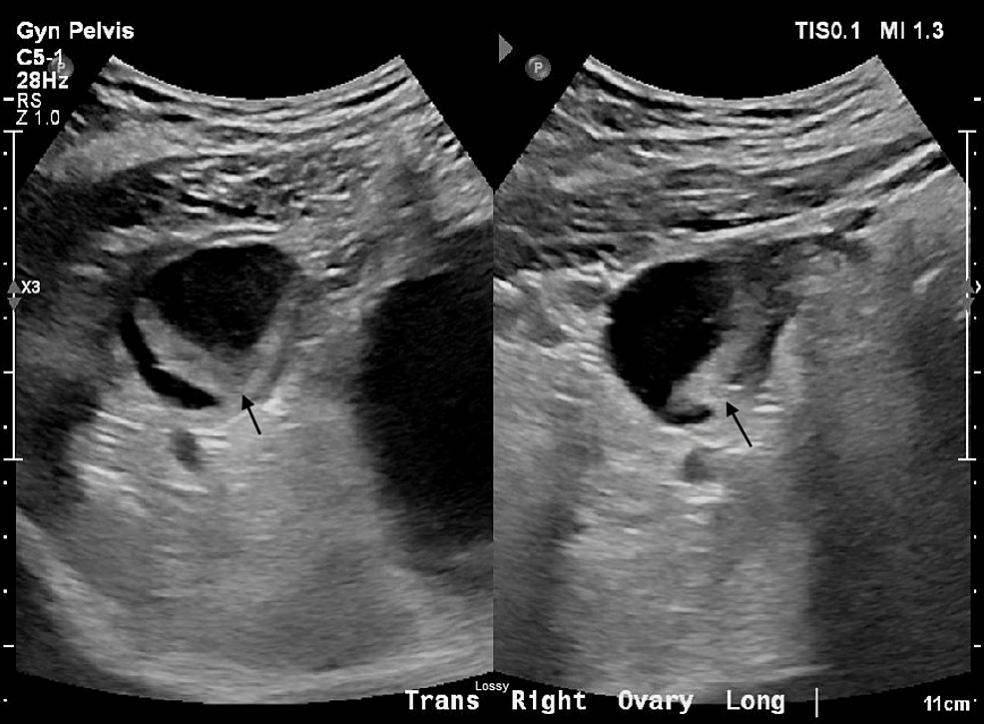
Right Ovarian Heterogeneity on Transvaginal Ultrasound Arrows indicate areas of heterogeneity
